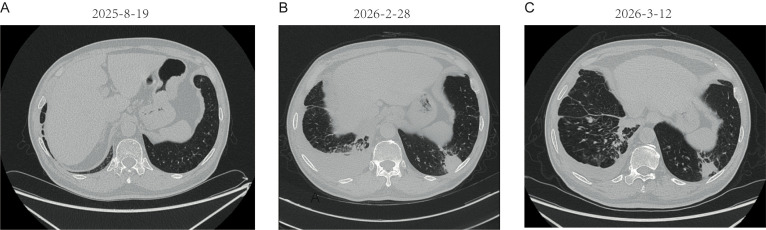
Chest CT findings. (A) No soft-tissue lesion was detected in the posterior basal segment of the left lower lobe on August 19, 2025. (B) A newly detected soft-tissue lesion was seen in the posterior basal segment of the left lower lobe on February 28, 2026. (C) Marked shrinkage of the left lower lobe lesion was observed on follow-up CT obtained on March 12, 2026.
